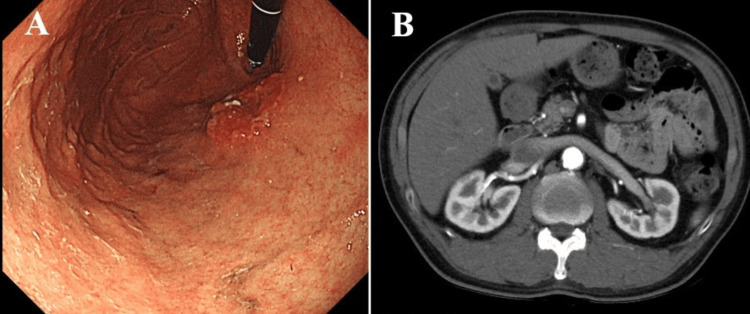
Gastrointestinal endoscopy and contrast-enhanced CT findings. (A) Gastrointestinal endoscopy revealed an advanced gastric tumor measuring 30 × 30 mm in the anterior wall of the cardia. (B) Contrast-enhanced CT showed no enlarged LN or distant metastasis. CT, computed tomography; LN; lymph node
